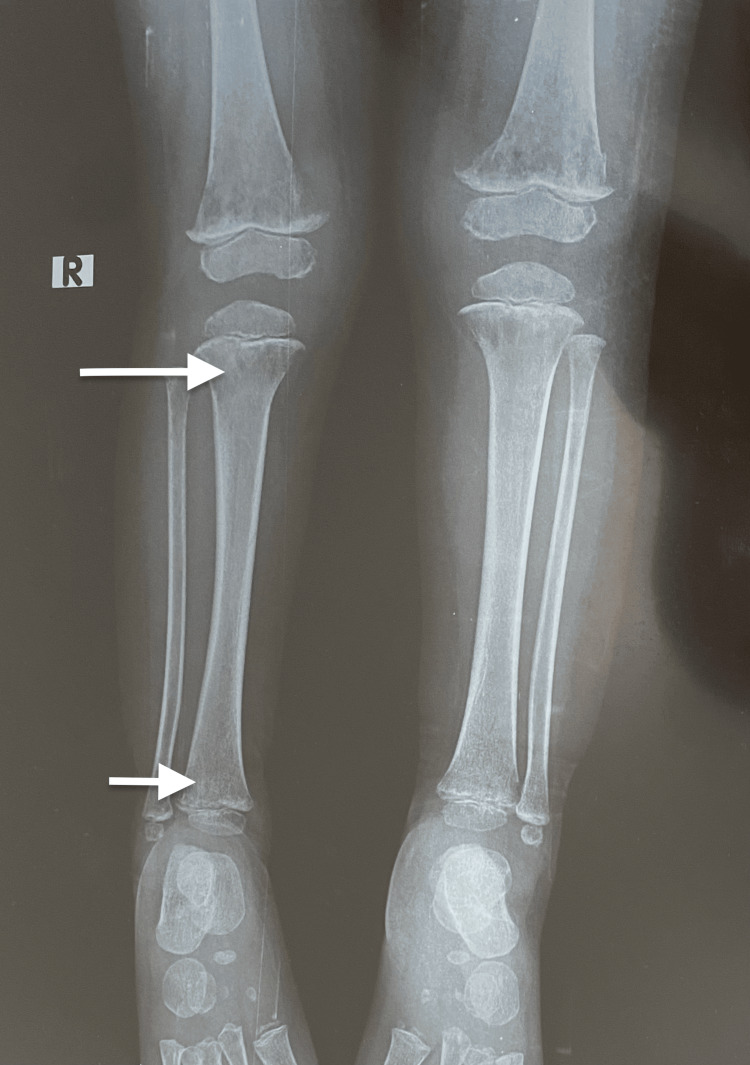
Plain radiograph of the lower limbs Diffuse bone rarefaction, bilateral osteolytic lesions of the tibias and fibulas, periosteal reaction, and metaphyseal radiolucent bands (“leukemic lines”) at the knee and ankle regions (arrows), consistent with leukemic bone infiltration. No pathological fracture is identified
